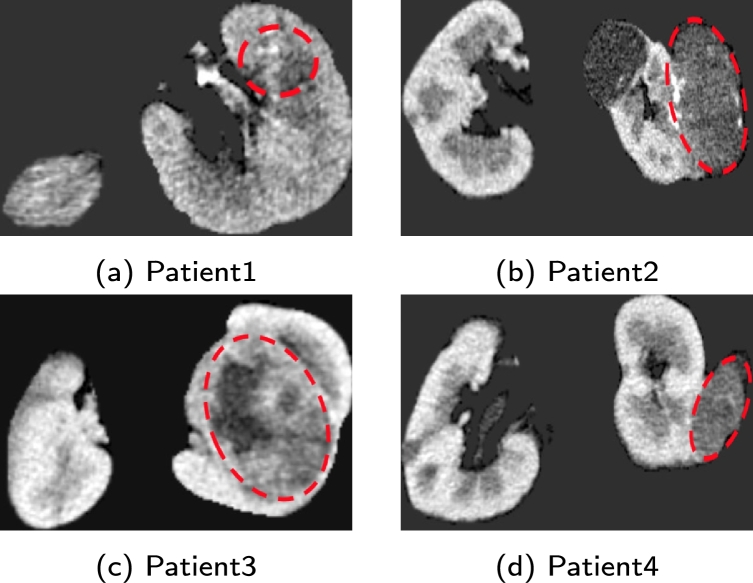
CT images with tumors indicated of patients with event 1.
